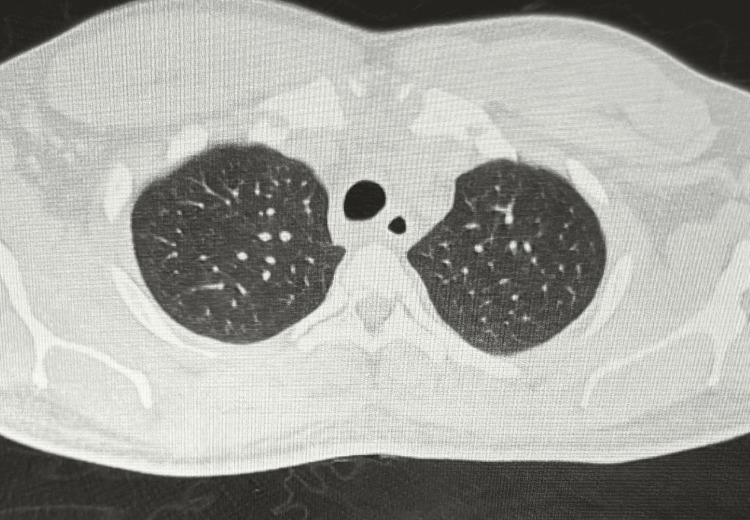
CT with contrast performed to rule out oesophageal perforation CT: computed tomography
